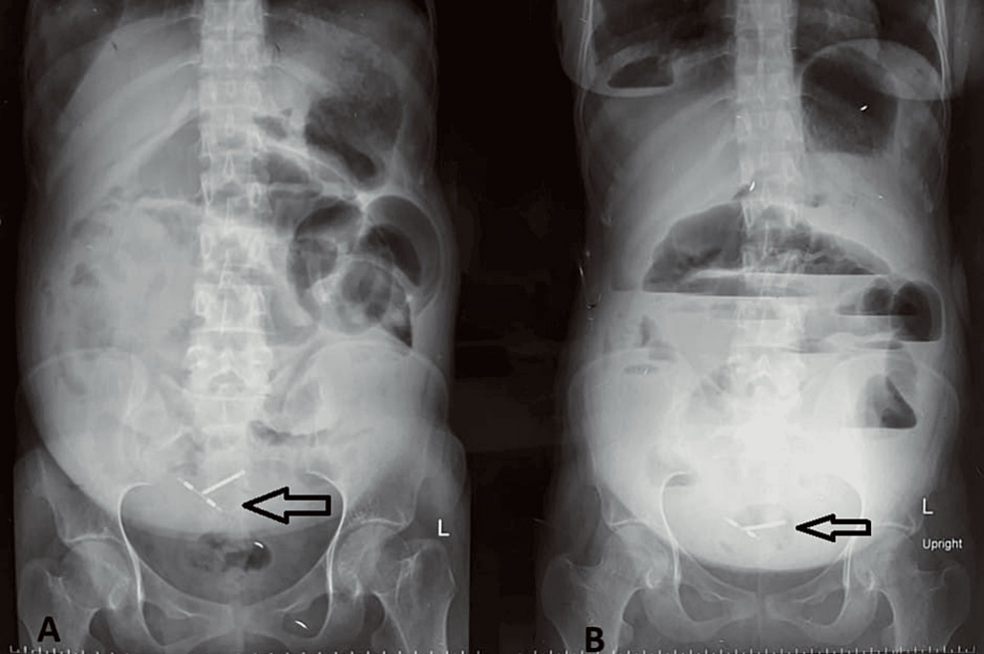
(A) Pre-operative plain radiograph of the abdomen showing copper T (black arrow) in the pelvis with multiple air-fluid levels. (B) Plain radiograph of the abdomen in supine position showing dilated bowel loops.
